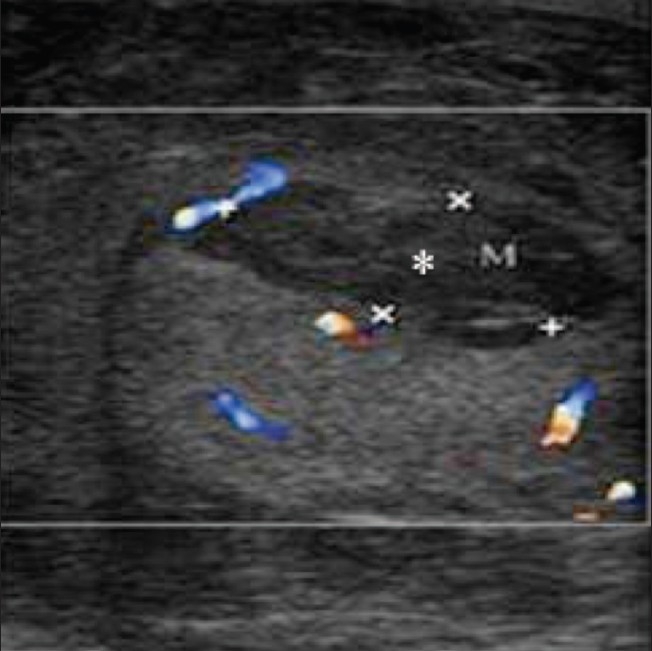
Testicular trauma. Color Doppler shows no color flow in the focal lesion (asterix) due to trauma
